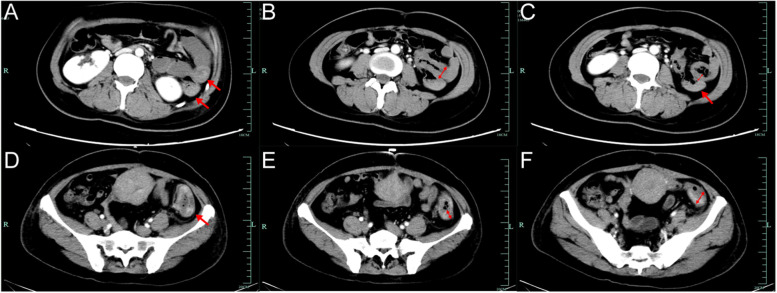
The whole-abdomen CT images: lesions in the intestine presented as thickening of the bowel wall and blood in the intestine presented as slightly dense shadows in the intestine. Patient A: A a slightly dense shadow in part of the small bowel and colorectal lumen (red arrow), B a slightly thickened small bowel wall in part of the left mid abdomen in the coronal section (red double arrow), and C a slightly thickened small bowel wall in the transverse section (red double arrow) and a slightly dense shadow in part of the small bowel (red arrow). Patient B: D thickening of the bowel wall in the lower part of the descending colon (red arrow), E long diameter of the thickened portion of the bowel (red double arrow), and F transverse diameter of the thickened portion of the bowel (red double arrow)
